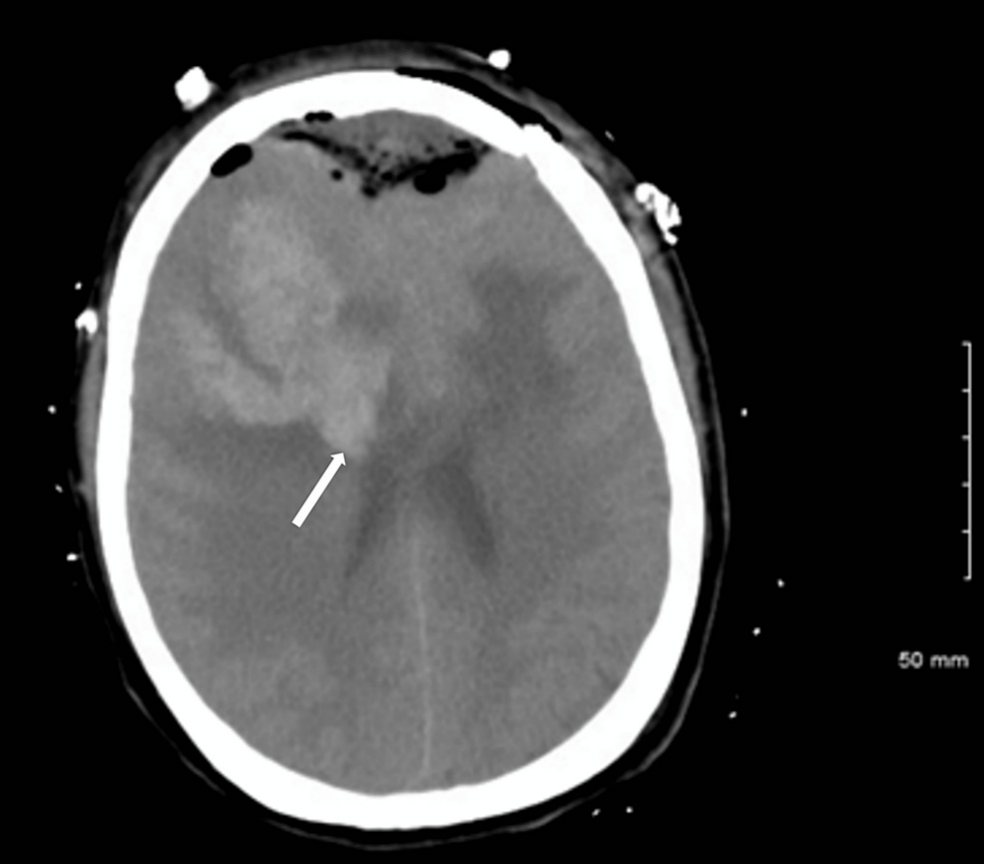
MRI of the brain showing the abnormal appearance of the anterior third of the superior sagittal sinus
